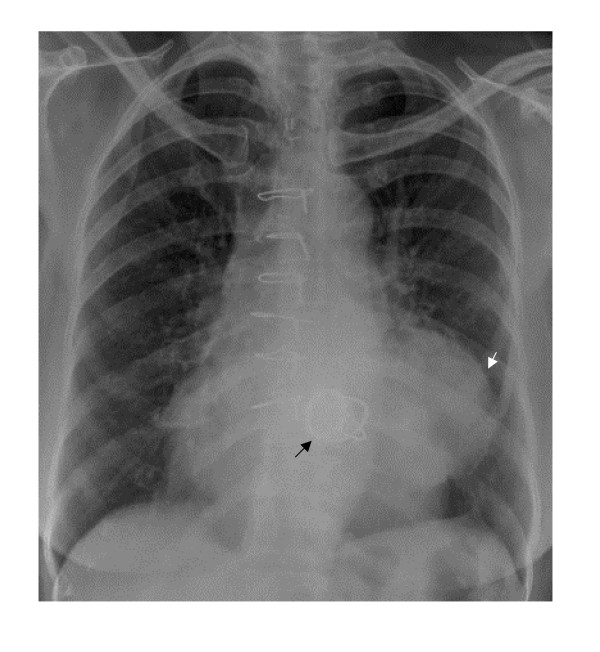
Chest radiograph in posteroaanterior view showed the rounded opacity along left cardiac silhouette.
